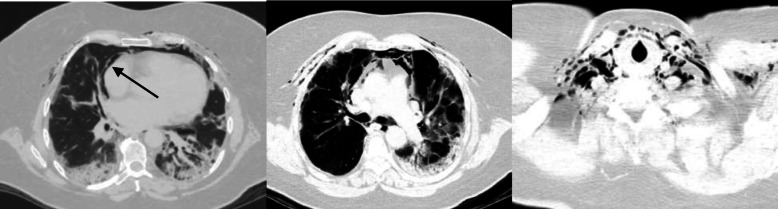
Computed tomography of the chest without contrast of Patient 1. There is a pneumopericardium (left) and extensive pneumomediastinum (middle) extending along soft tissues of the anterior and lateral chest wall as well as anterior neck soft tissues (right)
